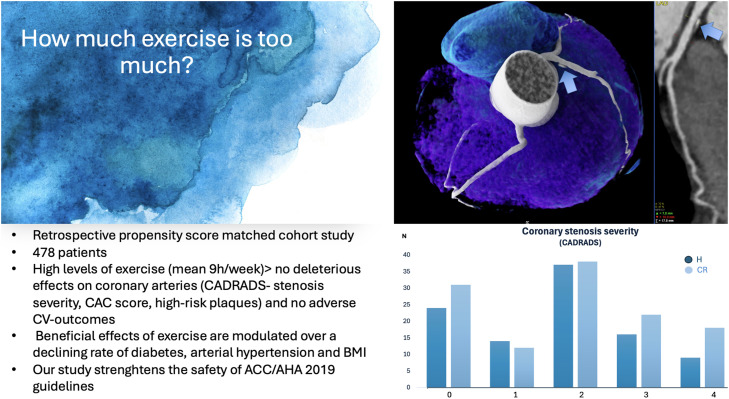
The influnece of high exercise levels on coronary atherosclerosis by coronary computed tomography angiography.
